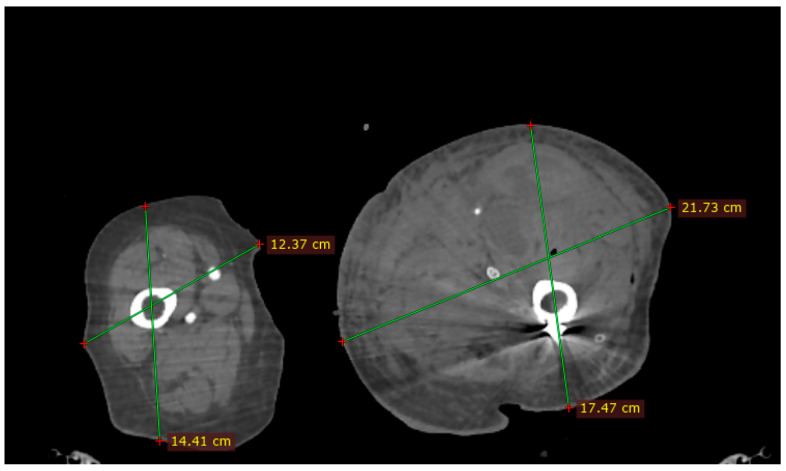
Angio CT image: Dimensional difference between left and right thigh.
